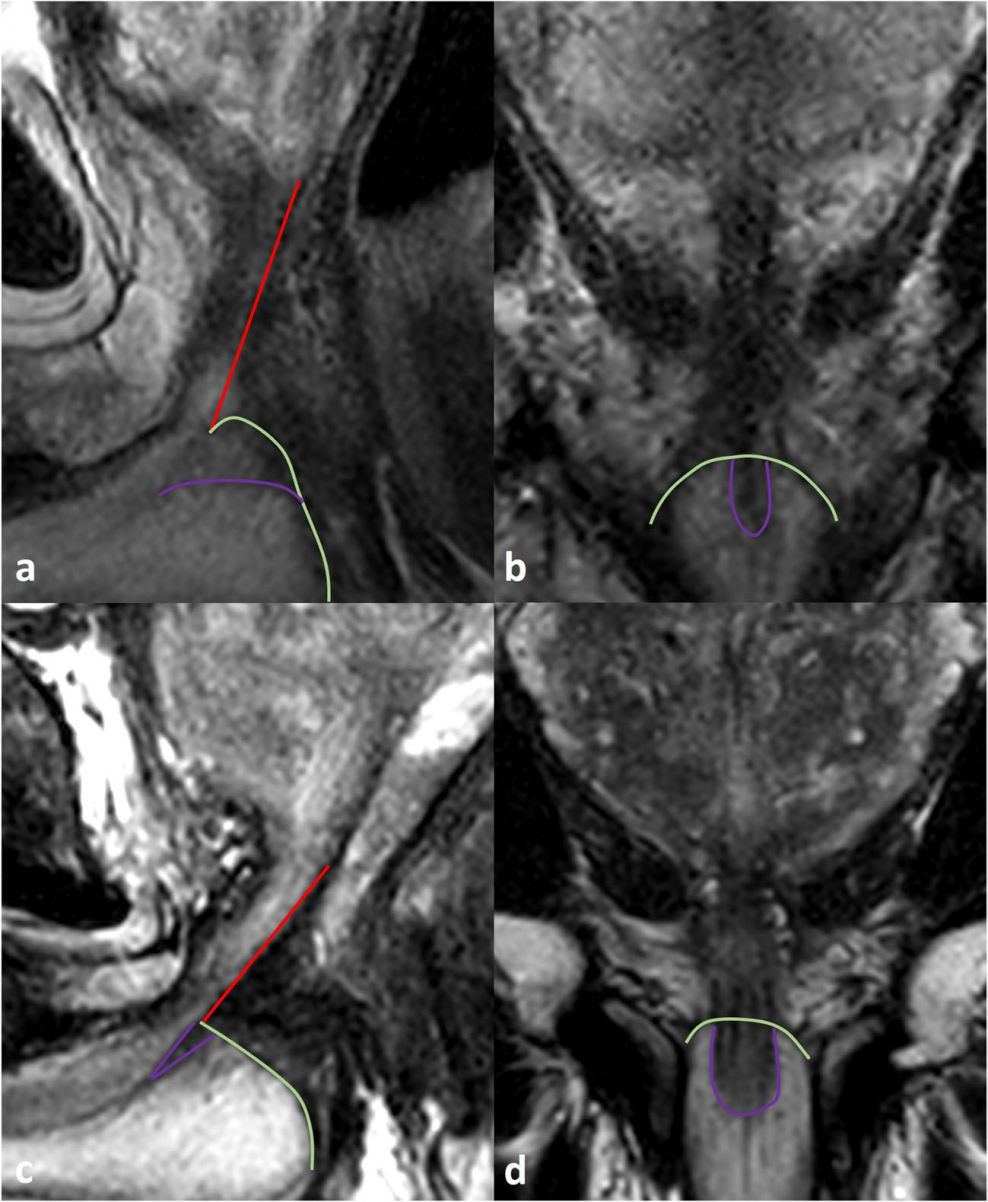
Pitfall in membranous urethral length (MUL) measurement—the lower border, intersecting with the penile bulb (2). The upper contour of the penile bulb may sometimes be difficult to determine at the midsagittal image. Peribulbic tissue surrounding the membranous urethra has various low T2 signal intensities. This tissue contains the perineal body, Cowper glands, and deep transverse perineal muscle. These structures are difficult to appreciate separately from the rhabdosphincter and similar dark signal intensity may continue several millimetres into the penile bulb. This may decrease the accurate demarcation of the lower border of the MU, resulting in extended MUL measurement with poor reproducibility. Scrolling 1 or 2 slices parasagittal (and when difficult) crosslinking with the coronal will help to identify the lower limit of the membranous urethra correctly. a A prostate cancer patient with sagittal T2w images on preoperative MRI. The proposed MUL measurement (red line) was challenging at the lower border, at the intersection with the penile bulb (green line). A ‘double contour’ appearance was suggested on the midsagittal image, as a result of partial volume effects (purple line). b Scrolling through the sagittal images left and right (not shown) and crosslinking with coronal images determined the appropriate upper contour of the penile bulb (green) and the intrabulbic hypointense tissue (purple line). c A prostate cancer patient with sagittal T2w images on preoperative MRI. The proposed MUL measurement (red line) was challenging at the lower border, at the intersection with the penile bulb (green line), due to the intrabulbic continuation hypointense tissue surrounding the urethra at the midsagittal image. d Scrolling through the sagittal images left and right (not shown) and crosslinking with coronal images determined the appropriate upper contour of the penile bulb (green) and the intrabulbic continuation of hypointense tissue surrounding the urethra (purple line)
